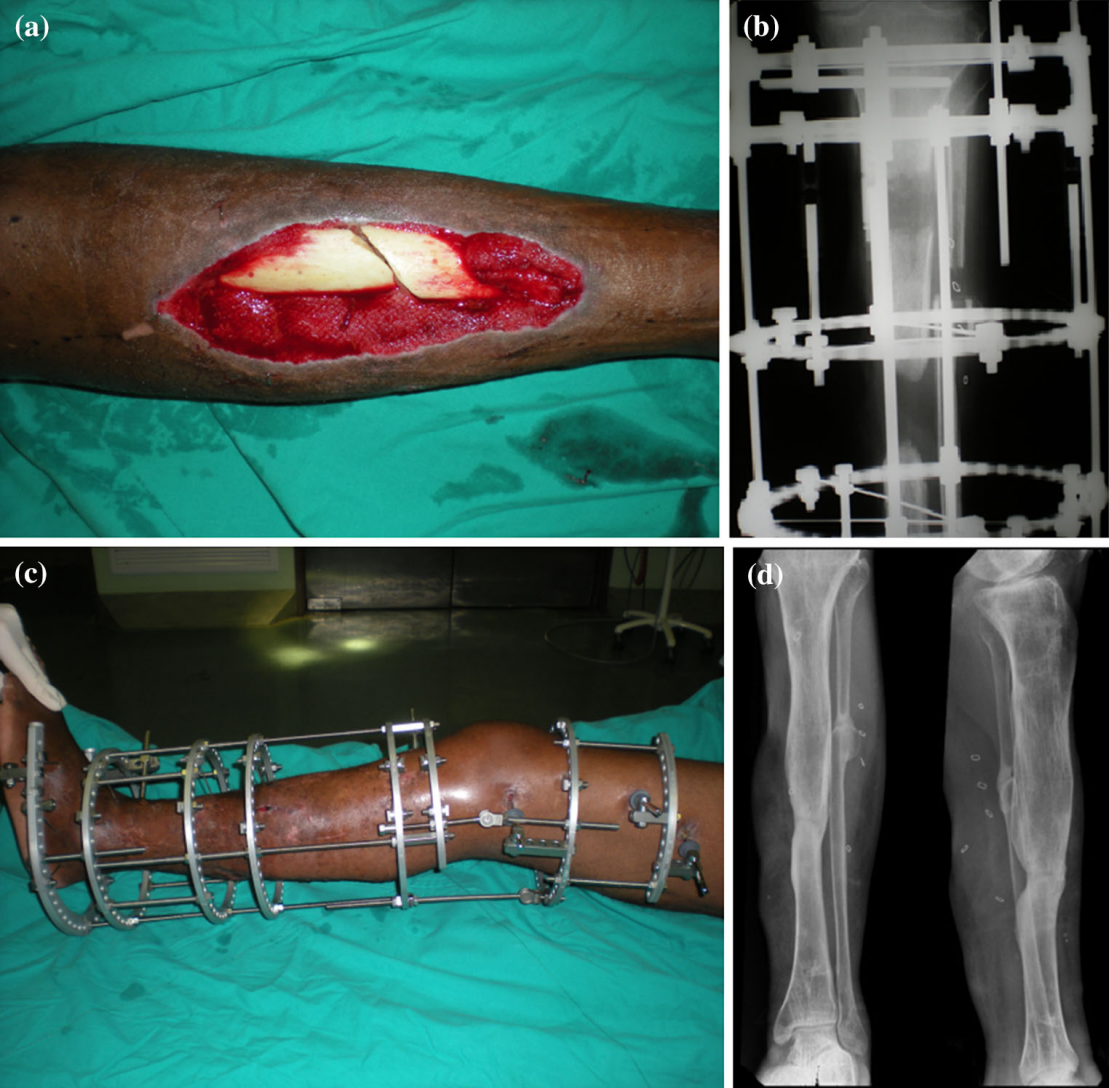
Clinical and radiological features of a case complicated by knee flexion and equinus contractures. a Wound dehiscence following open reduction and intramedullary nailing of a neglected tibia fracture. b Distraction osteogenesis following removal of the PMMA spacer. c Gradual correction of the knee and ankle deformities. d Final radiographs showing satisfactory consolidation of the regenerate and union at docking site
